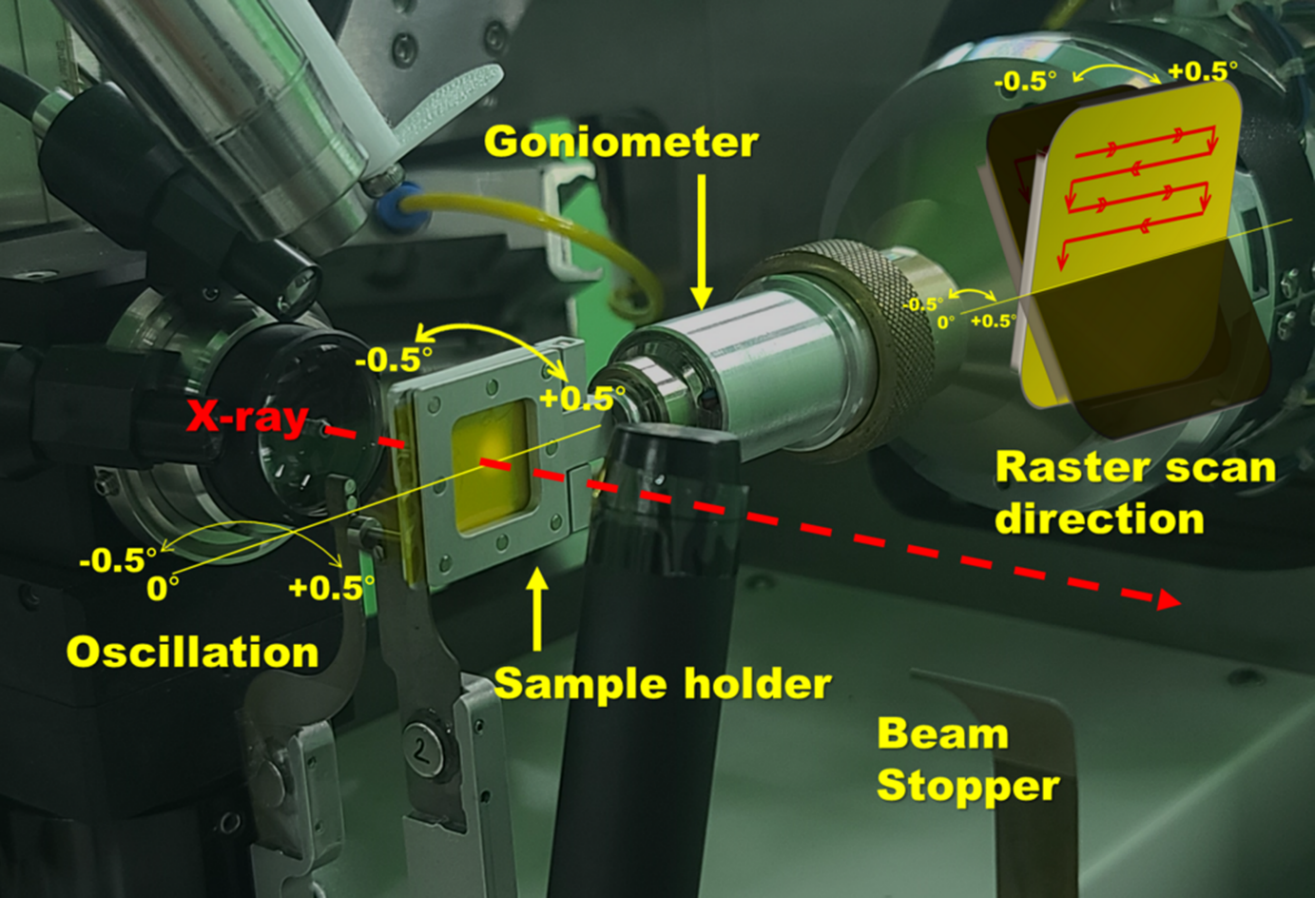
Photograph of the flip-holder mounted on the BL11C beamline (Park *et al.*, 2017 ▸). The X-ray beam is indicated by the red dashed arrow. The sample holder and goniometer are displayed by yellow arrows. The center line of oscillation is displayed by a yellow line. The raster scan direction is displayed by red arrows.
